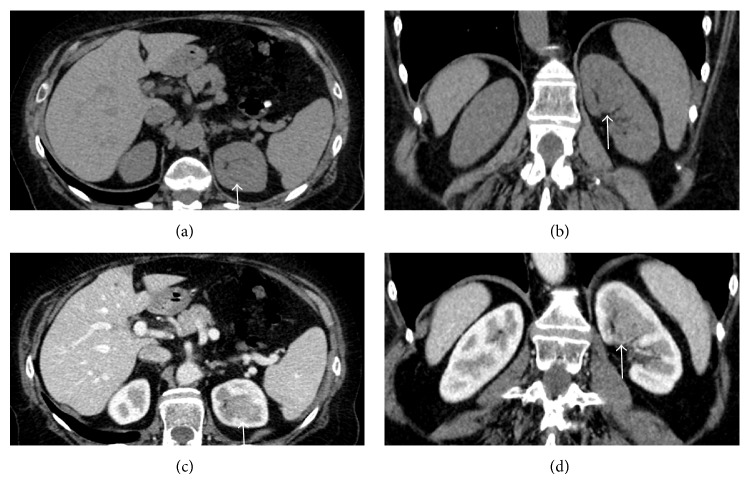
Papillary carcinoma. ((a)-(b)) Unenhanced CT scans. ((c)-(d)) Contrast-enhanced CT scans. A mass with a modest enhancement after contrast medium injection is evident at the upper portion of the left kidney (arrows).
